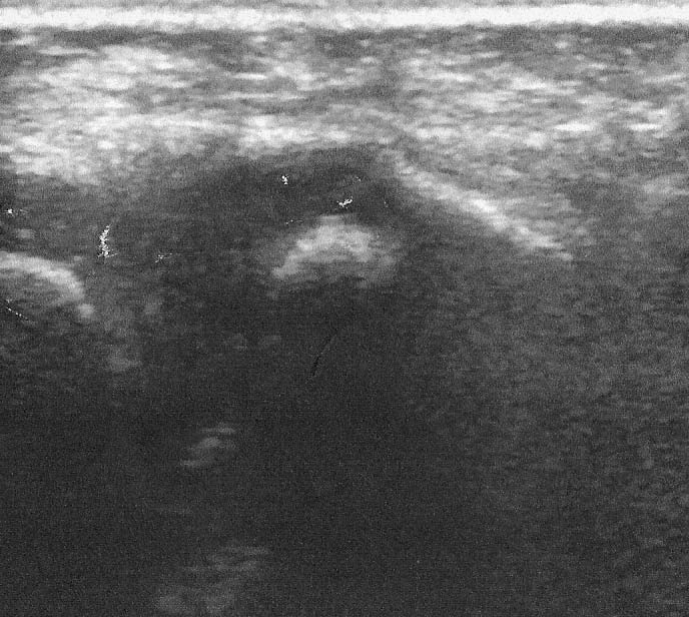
Case 2- The Ultrasound verifies the absence of recurrence .
